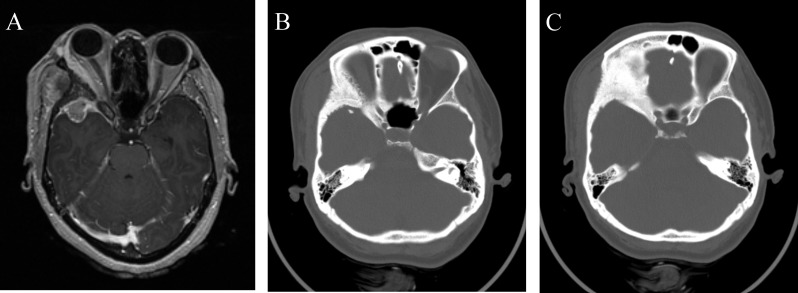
Preoperative MRI and CT imaging of right middle fossa meningioma (A) Axial T1-weight MRI with contrast revealing a right middle fossa meningioma with associated hyperostosis of surrounding bone and invasion of the temporalis muscle. (B and C) Extensive bony hyperostosis of the right lateral orbit and pterion.
